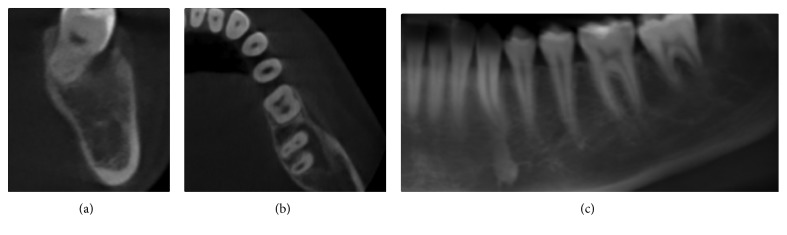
8-month radiographic examination: CBCT panoramic reconstruction, showing partial healing of the lesion and normal eruption of 37 (a); axial cut showing partial healing of the lesion and almost normal position of 37 (b); cross-sectional view at the level of 37 showing partial healing of the lesion (c).
